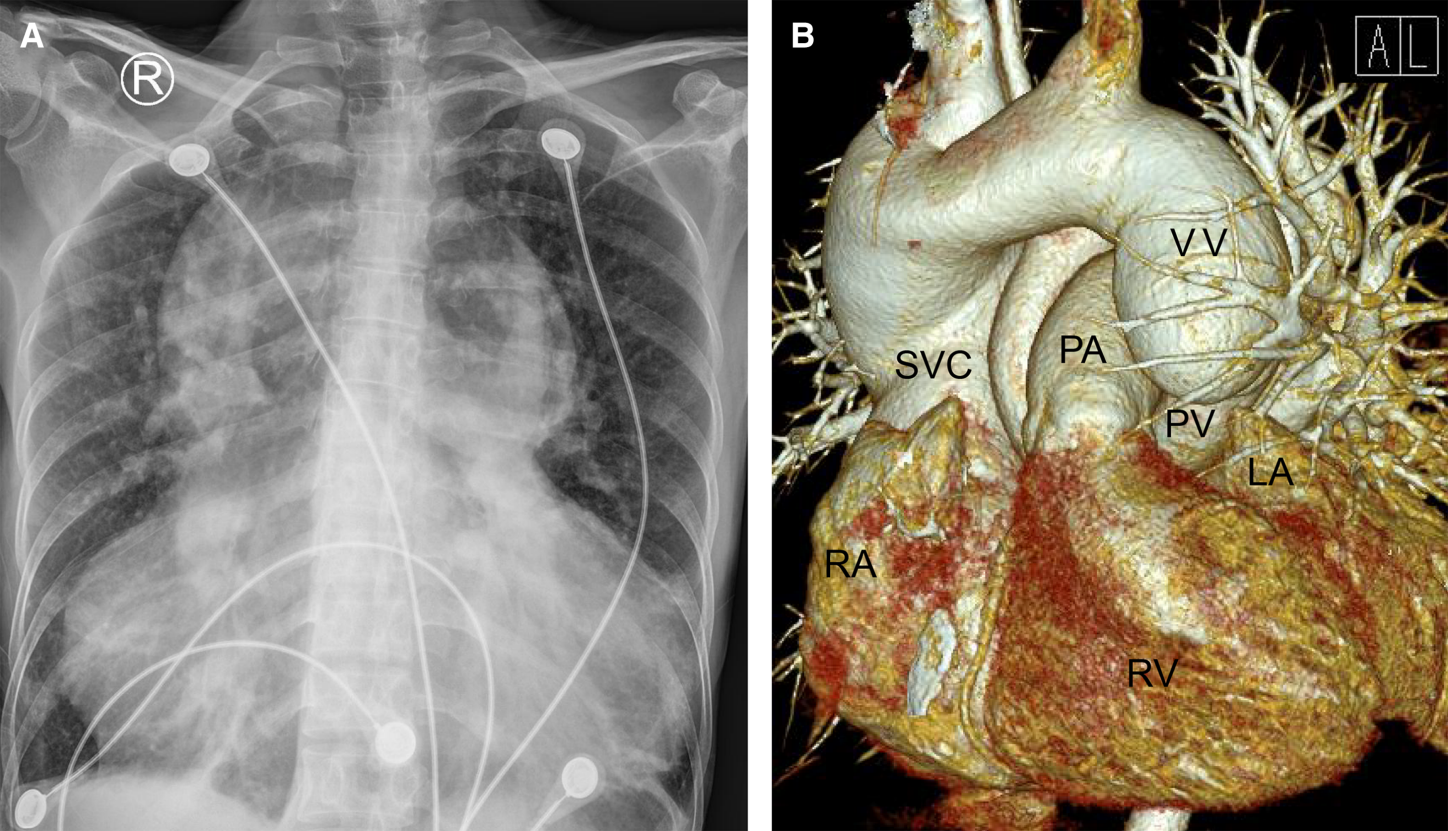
Chest radiography (A) and cardiac computered tomograhy angiography (B) preoperatively indicated that the lungs were clearly textured, the heart shadow was significantly enlarged (A); the left and right pulmonary veins were not connected to the left atrium but merged into a vertical vein and drained upward into the left brachiocephalic vein, innominate vein and then the superior vena cava. (B) LA, left atrium; PA, pulmonary artery; PV, pumonary vein; RA, right atrium; RV, right ventricle; SVC, superior vena cava; VV, vertical vein.
